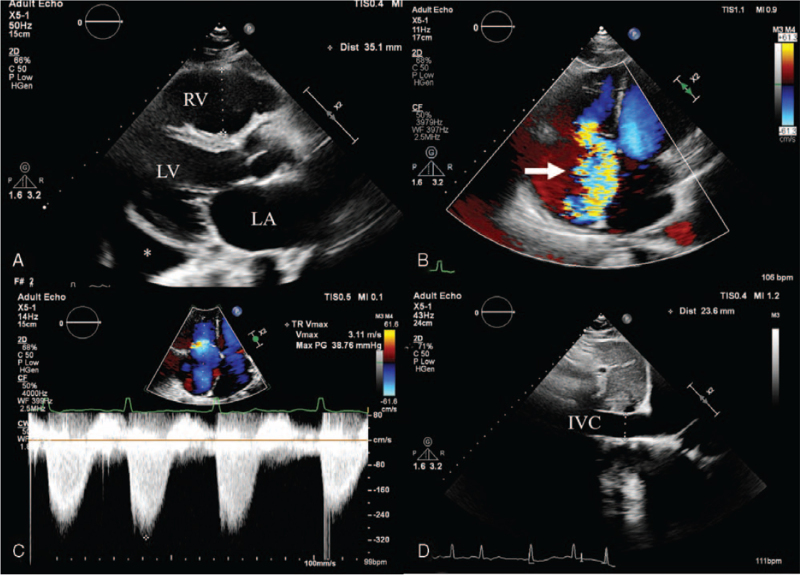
Transthoracic echocardiography at the time of diagnosis of severe tricuspid regurgitation (TR) showing (A) dilated right ventricle, (B) severe TR (arrow) on a color Doppler image, and (C–D) moderately increased pulmonary artery systolic pressure of 59 mmHg with inferior vena cava plethora. IVC = inferior vena cava, LA = left atrium, LV = left ventricle, RV = right ventricle; asterisk = pleural effusion.
